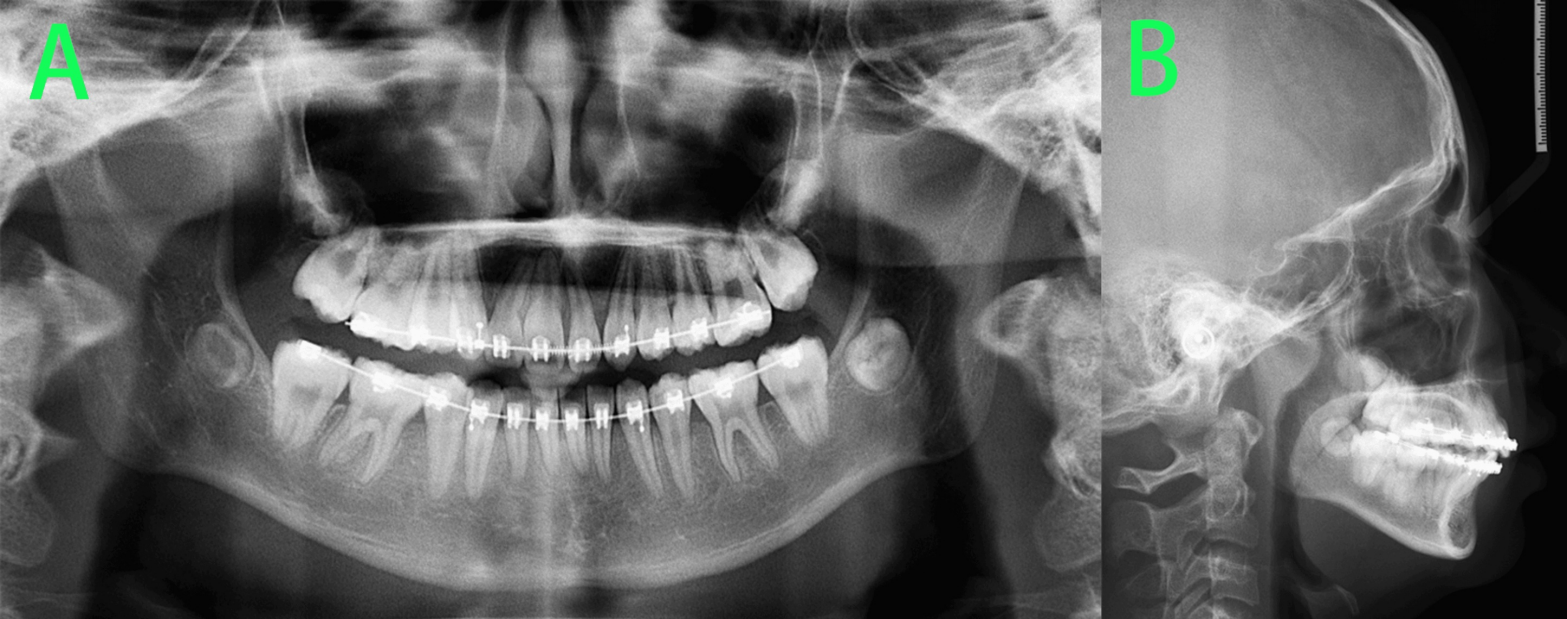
Post-treatment radiographs A: The pre-debonding panoramic radiograph indicated no significant root or alveolar bone resorption in the permanent dentition. B: The lateral cephalometric radiograph revealed an improvement in the skeletal Class II pattern compared to pretreatment, although mandibular retrusion persisted, the maxillary anterior teeth were significantly retracted.
